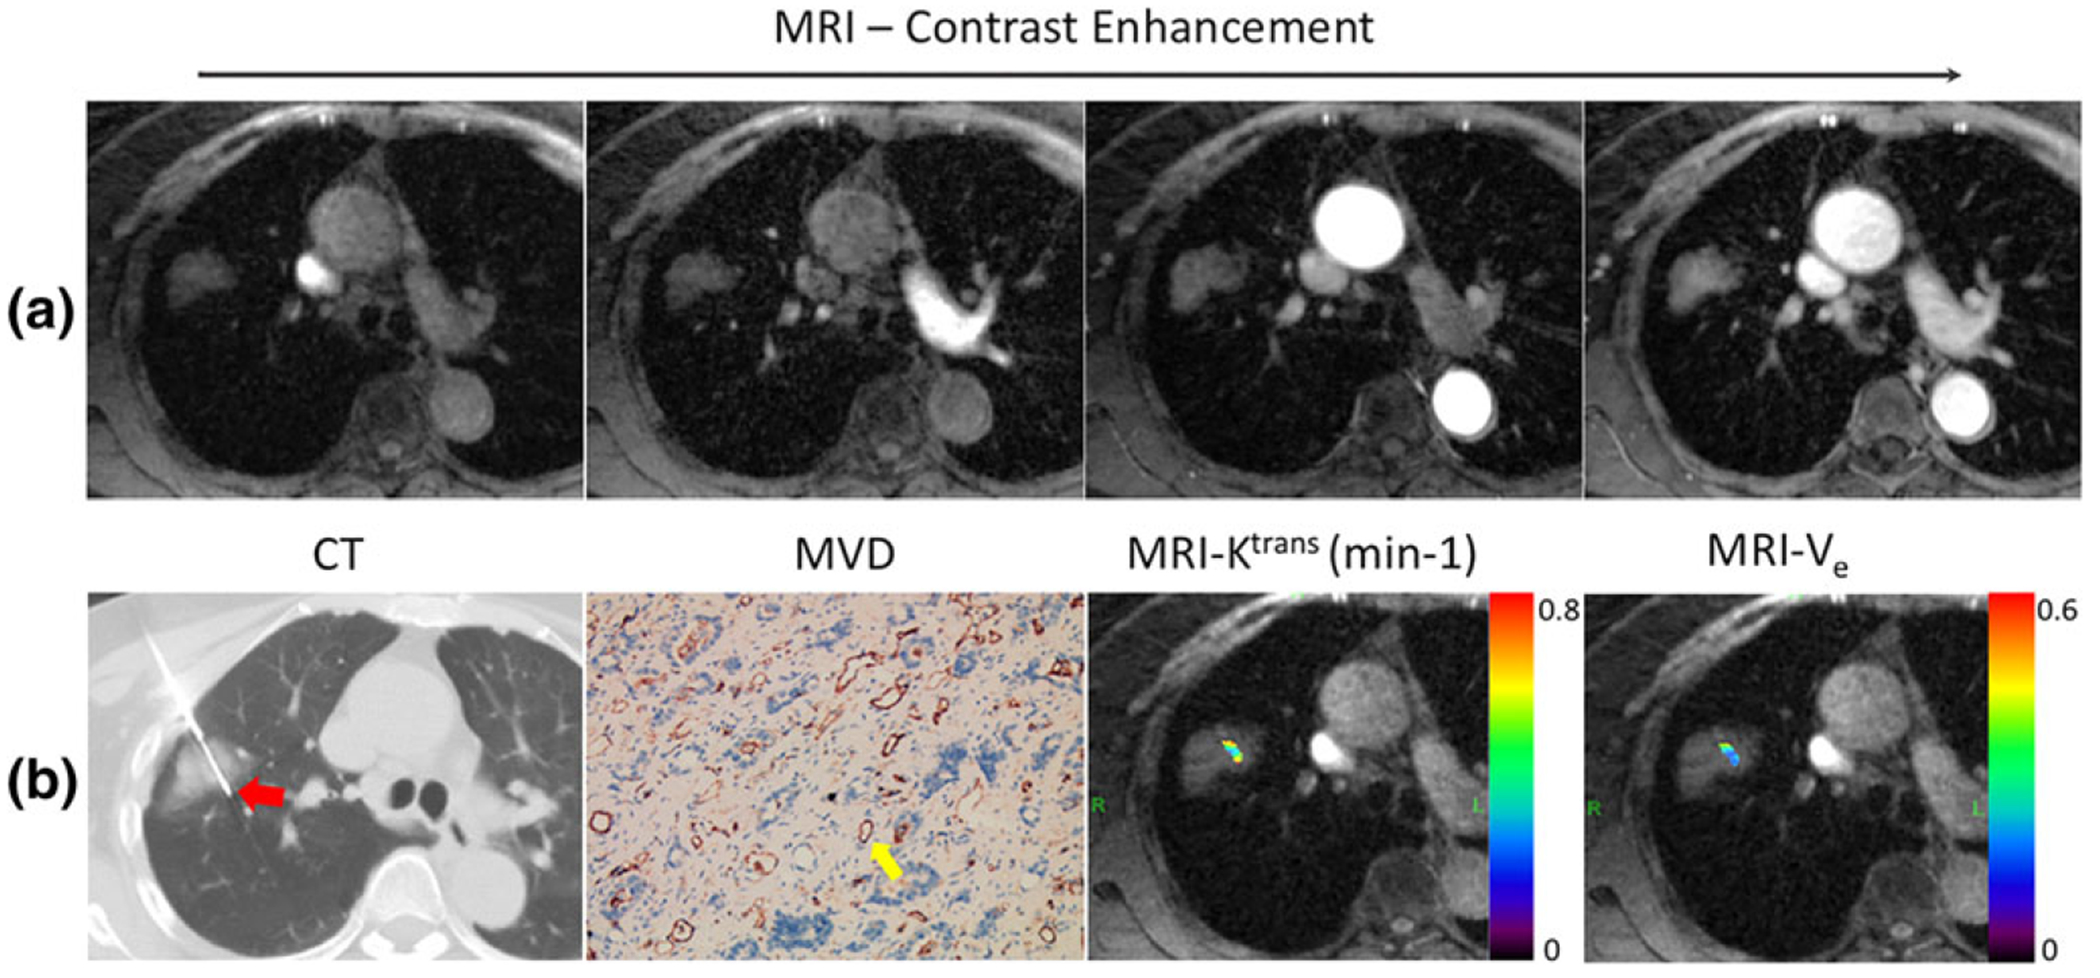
(a): Four different GRASP contrast phases from a patient with adenocarcinoma. (b): Corresponding CT-biopsy image, MVD image, and regional K^trans^ and V_e_ maps superimposed on a corresponding GRASP image. The yellow arrow indicates a microvessel and the red arrow indicates the needle in CT biopsy.
